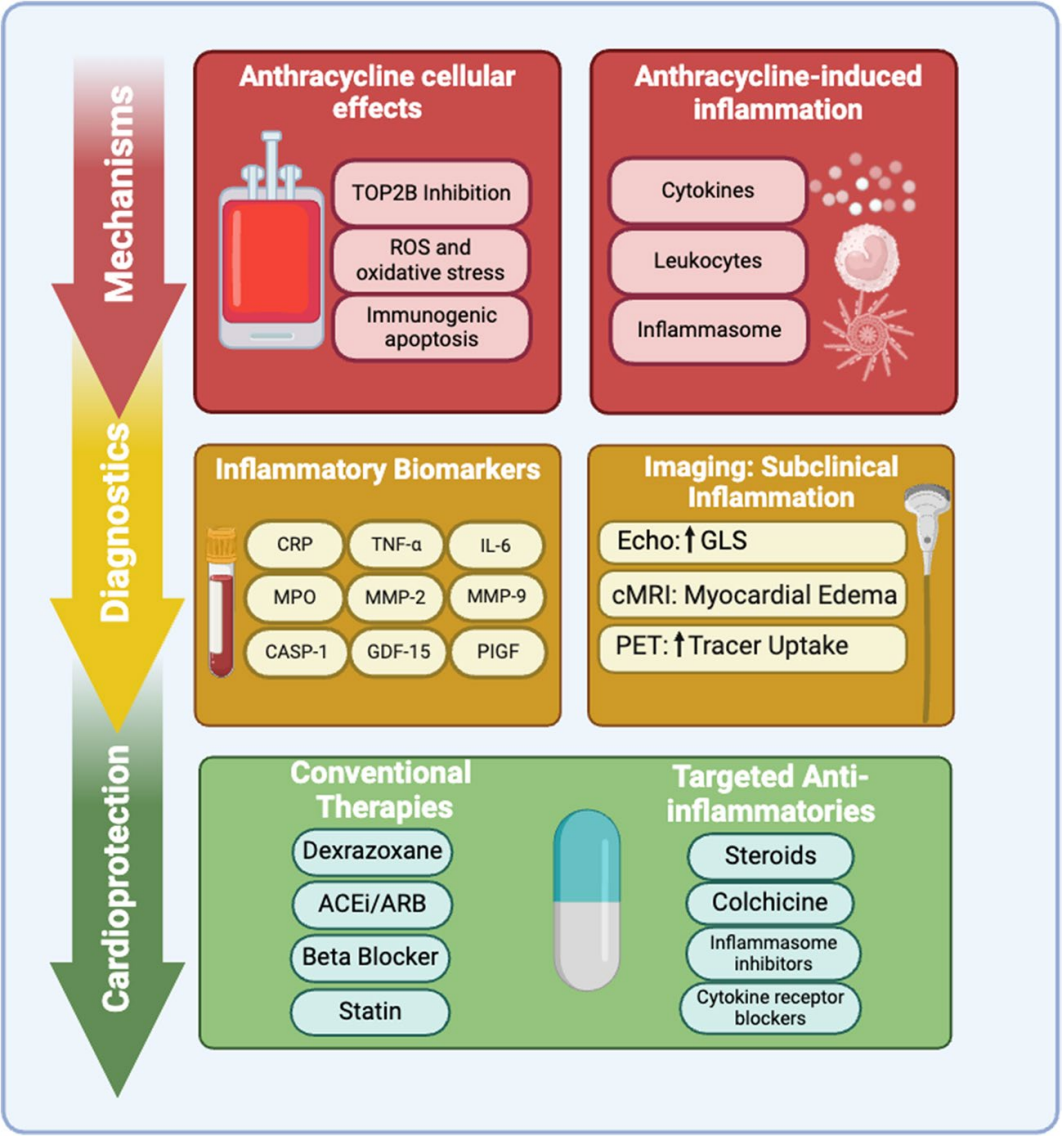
Anthracycline-induced Inflammation: Mechanisms, Diagnostics, Cardioprotection.  Abbreviations: TOP2B: Topoisomerase II beta; ROS: Reactive Oxygen Species; CRP: C-reactive Protein; TNF-α: Tumor Necrosis Factor-alpha; IL-6: Interleukin-6; MMP-2: Matrix Metalloproteinase-2; MMP-9: Matrix Metalloproteinase-9; MPO: Myeloperoxidase; CASP-1: Caspase-1; GDF-15: Growth Differentiation Factor 15; PIGF: placental growth factor; GLS: Global Longitudinal Strain; cMRI: Cardiac Magnetic Resonance Imaging; PET: Positron Emission Tomography; ACEi: Angiotensin-Converting Enzyme Inhibitor; ARB: Angiotensin Receptor Blocker; NLRP3: Nucleotide-binding Oligomerization Domain-like Receptor Family Pyrin Domain Containing 3
